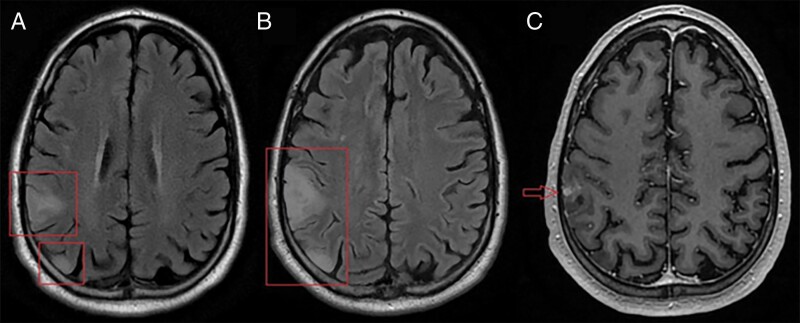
Axial magnetic resonance imaging. (A) T2 FLAIR imaging of 2 initial right parietal lobe masses. (B) T2 FLAIR imaging of the same masses, 3 months later. Both lesions infiltrate further into the parietal lobe and mild gyral swelling and effacement of adjacent sulci is seen. (C) Additionally, a nodular enhancing area is seen on the T1-weighted image.
